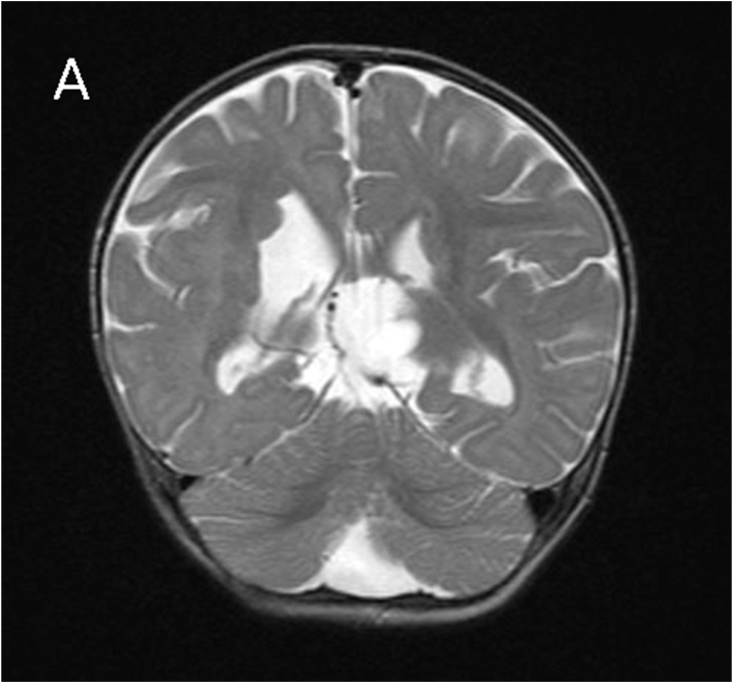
Magnetic resonance imaging (MRI) for our patient at the age of 7 months. A: coronal T2-weighted image showing subependymal grey matter heterotopia.
